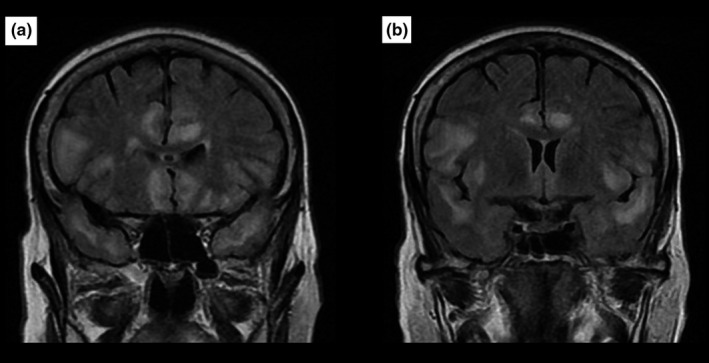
It is a 68‐year‐old woman without no past medical history, admitted for epileptic seizures associated with abnormal behavior and language disorders. *Brain MRI* shows on FLAIR sequences (*a* and *b*) bilateral hyperintensity in the temporo‐insular and cingulate regions. Diagnosis of anti‐NMDA‐R LE was made after the biological investigations
